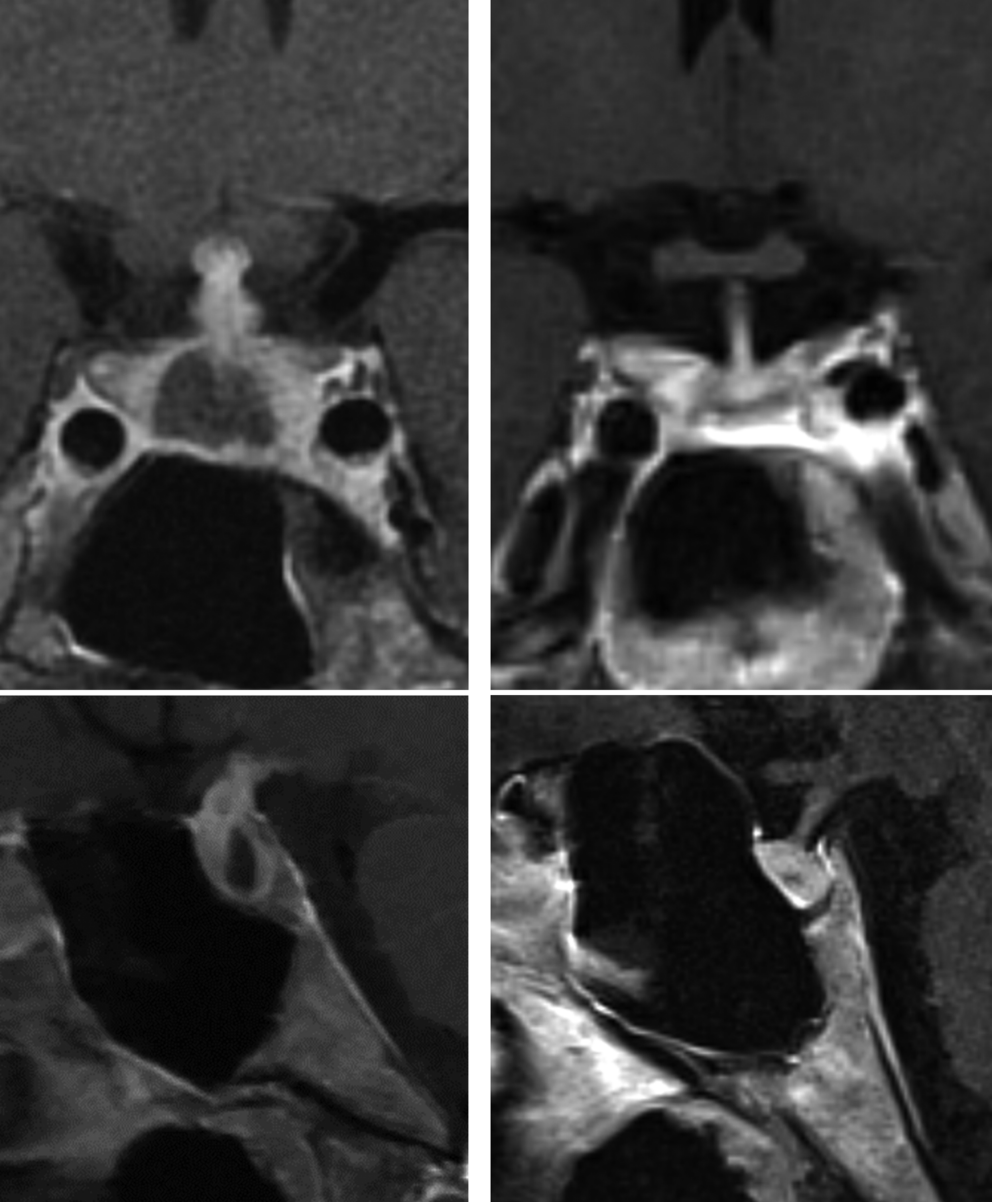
Lymphocytic hypophysitis—imaging. Postcontrast T1 pituitary magnetic resonance imaging coronal (top) and sagittal (bottom). Left, baseline. There is diffuse enlargement of the pituitary gland, with a central hypoenhancing area. There is also diffuse thickening and enhancement of the pituitary stalk and faint enhancement along the inferior margin of the hypothalamus. Right, 7 years after initial presentation, complete resolution of stalk thickening and gland enlargement.
